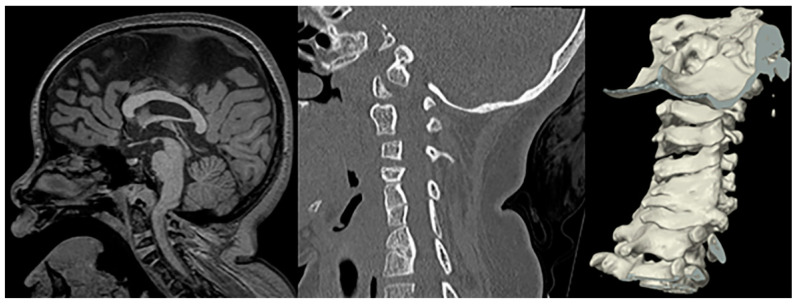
Sagittal T1-weighetd MRI and sagittal CT of the cervical spine including 3D surface rendering in a patient with confirmed Fanconi anemia show a narrowed foramen of magnum, mild impingement of the cranio-cervical junction, a short corpus callosum, and multilevel fusion anomalies of the cervical vertebral bodies.
